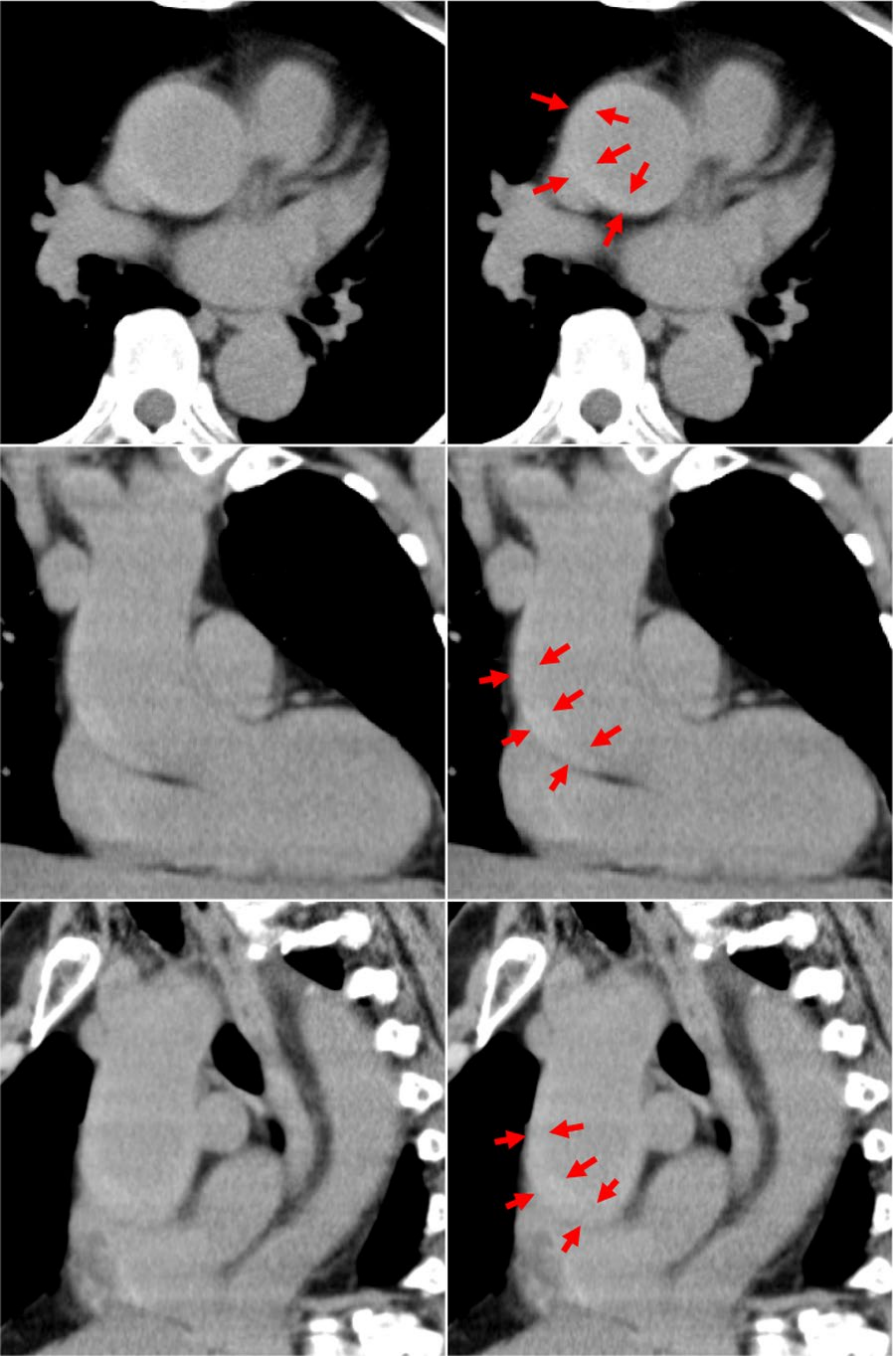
The axial, coronal, and sagittal views of the plain CT scan of case 1 from the top to the bottom. Note that difference in the contrast persisted throughout the ascending aorta (red arrows), indicating the presence of TAAAD with the thrombosed false lumen
